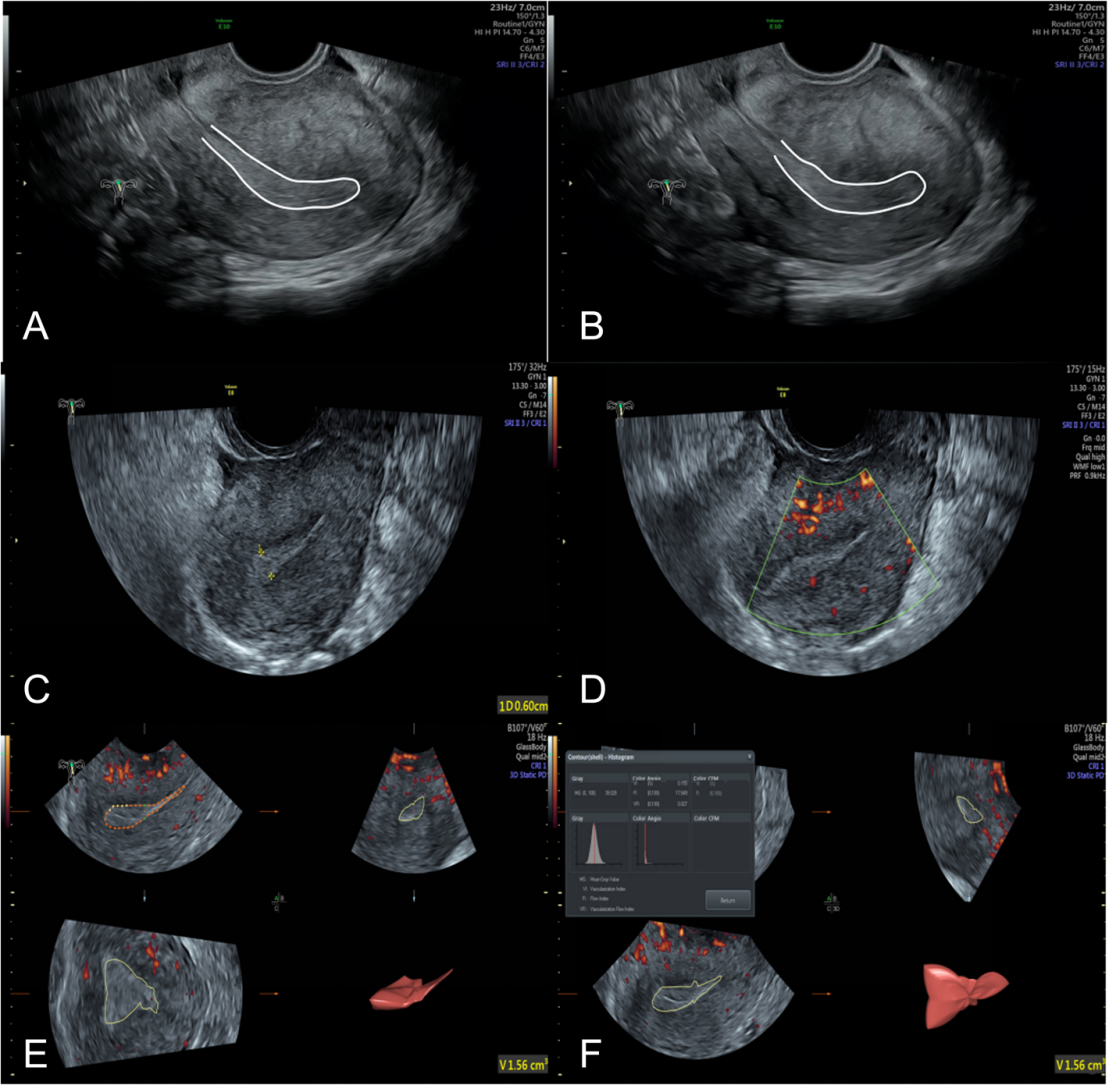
Ultrasound images of a subject in the AA group. a, b Two-dimensional gray-scale mode; two images during observation. White curve, outline of endometrium. No endometrium peristalsis wave within 3 min; score, 1; c two-dimensional gray-scale mode; endometrial thickness, 6.0 mm; score, 1; endometrial type, type C; score, 1; d 2D color Doppler mode; a little blood flow in the subendometrium; score, 2; e three-dimensional mode; EV, 1.56 cm^3^; score, 1; f three-dimensional mode; VFI, 0.027; score, 2
